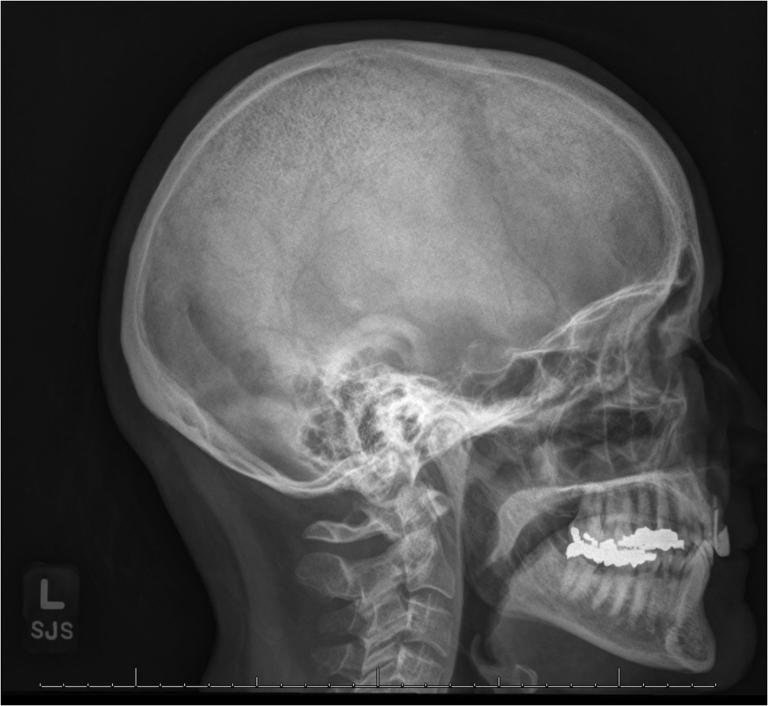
Radiograph of the lateral aspect of the calvarium. The radiograph of the lateral aspect of the calvarium demonstrates characteristic “salt and pepper” lesions with a granular appearance.
